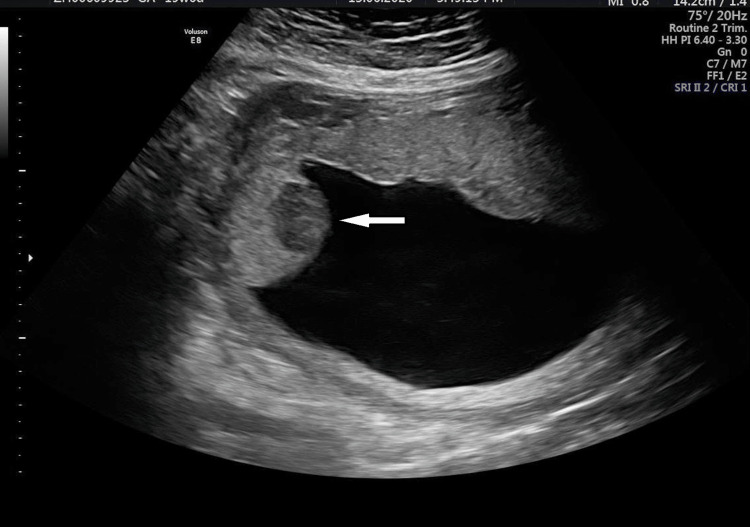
Ultrasound image at 20 weeks of gestation.
